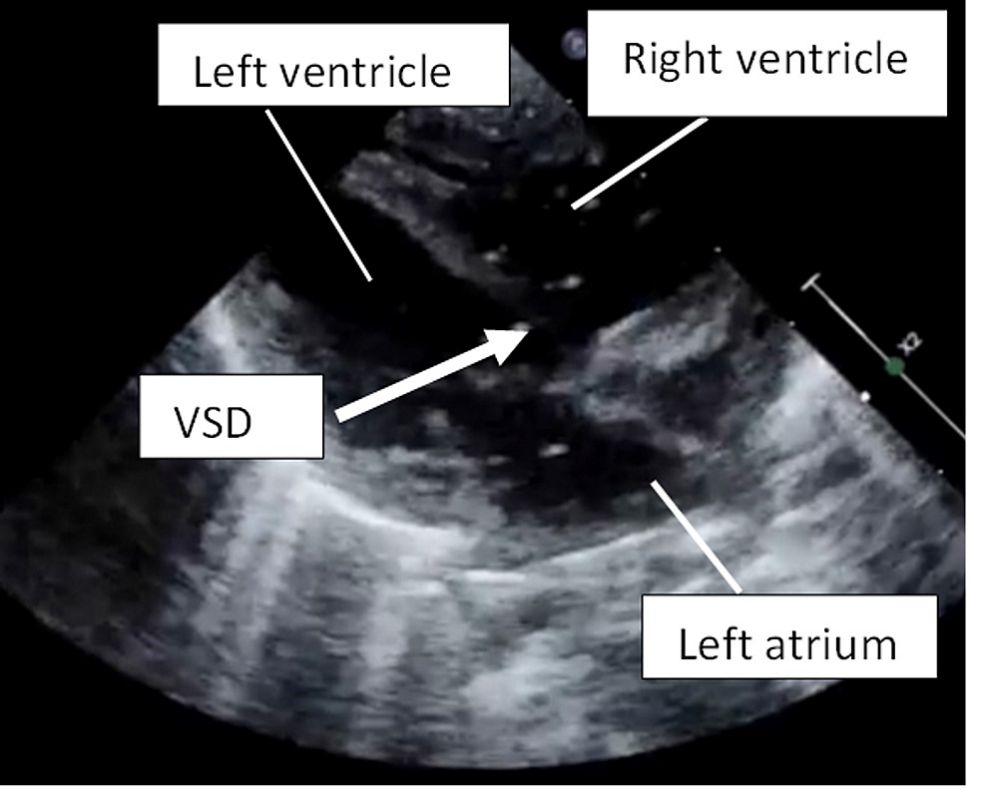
Bedside cardiac ultrasound (parasternal long axis) demonstrating air embolism (bright dots) going across the moderate ventricular septal defect (VSD). Bright dots: air embolism (air bubbles) Arrow: ventricular septal defect (VSD)
